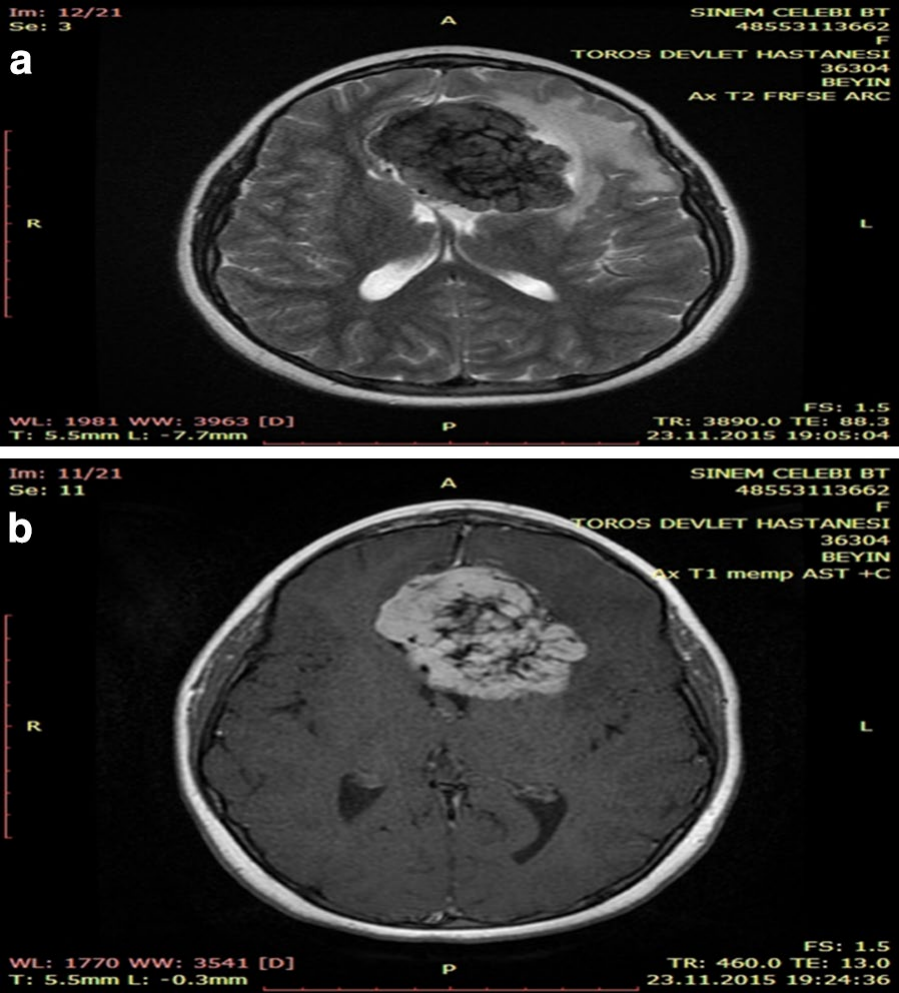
a, b Contrast-enhanced T2A and T1A MR images of the patient. Contrast uptake into the lesions is clearly detectable
